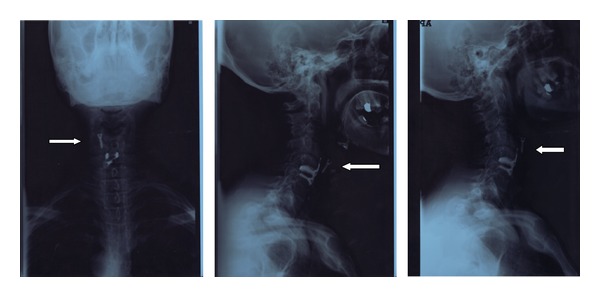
X-ray with contrast medium: the arrow clearly indicates the migration of the screw from the prosthesis in posteroanterior, right lateral, and left lateral projections.
